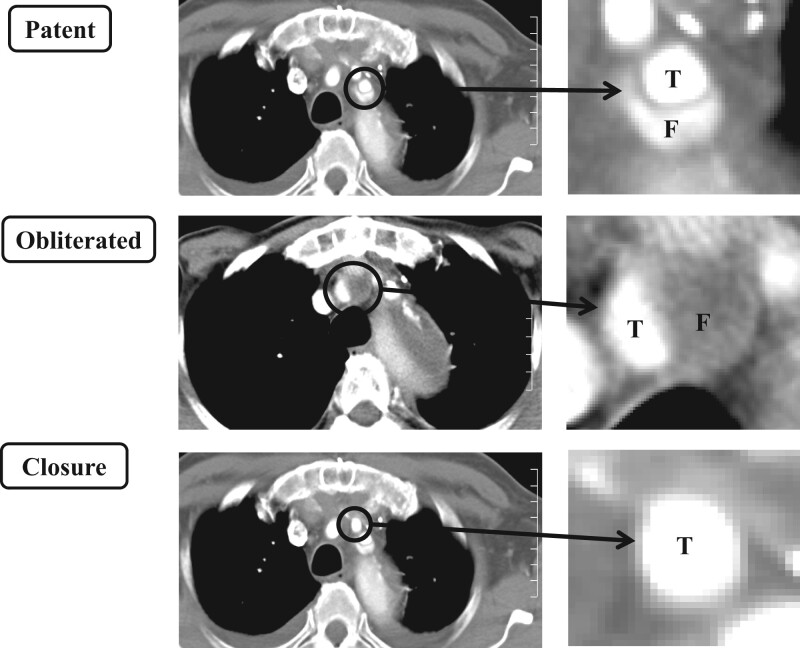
Three types of false lumen evaluated by computed tomography. Representative computed tomography images of patent, obliterated and closed false lumens.
